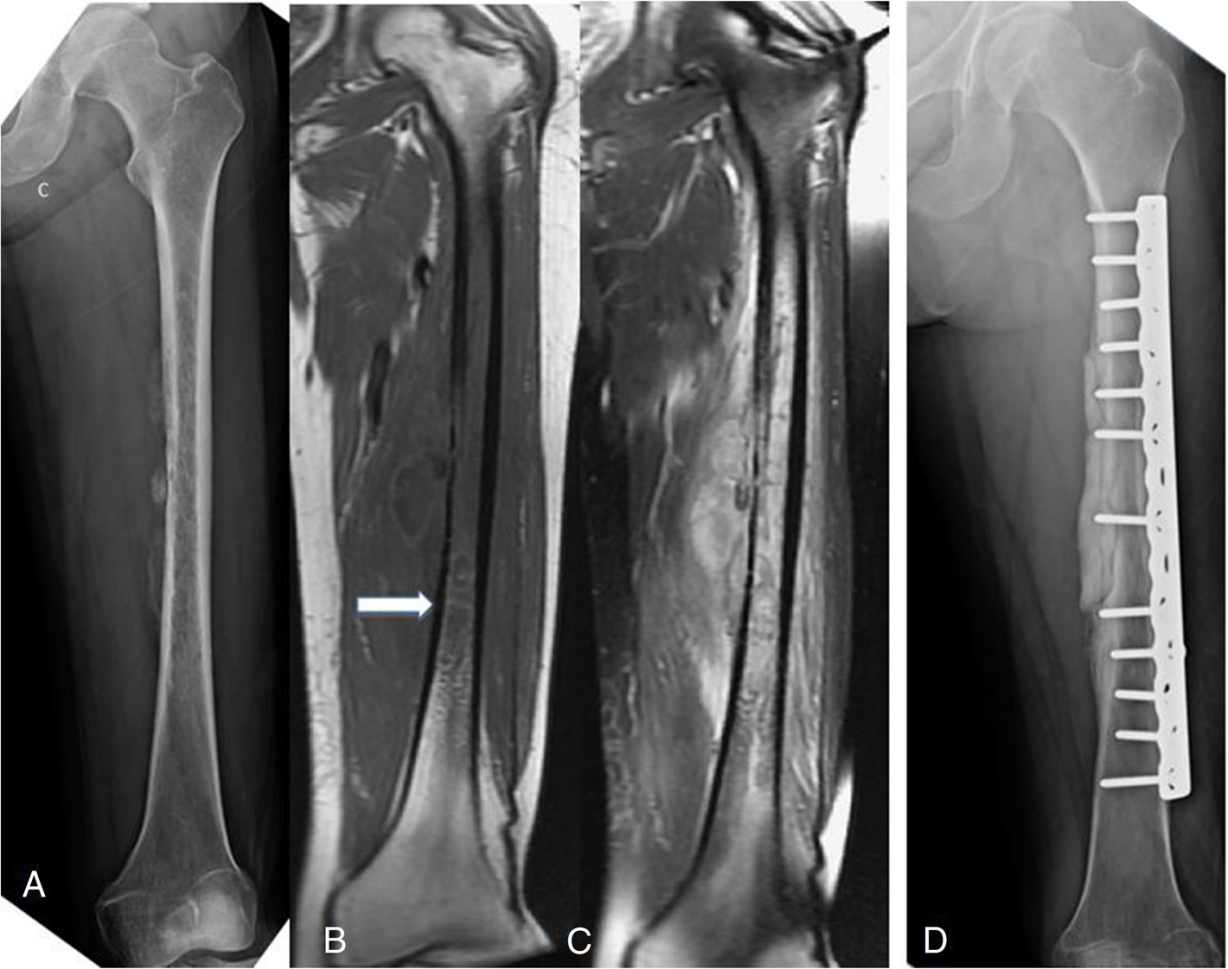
A 37-year-old woman with type 2 diabetes mellitus who complained of painful swelling of the thigh for one month. (A) A plain film showing a thin monolayer periosteal reaction with cortical destruction and moth-eaten osteolytic lesion. (B, C) Unenhanced T1-weighted magnetic resonance imaging (MRI) showing cystic lesion with a hyperintense granulation layer surrounding the central abscess cavity, and a T2-weighted image showing extensive soft tissue edema and lobulated abscess accumulation, representing the penumbra sign. (D) A plain film taken one year after the debridement and prophylactic ORIF surgery showing bony union and bone cement at the medial wall without signs of recurrence.
